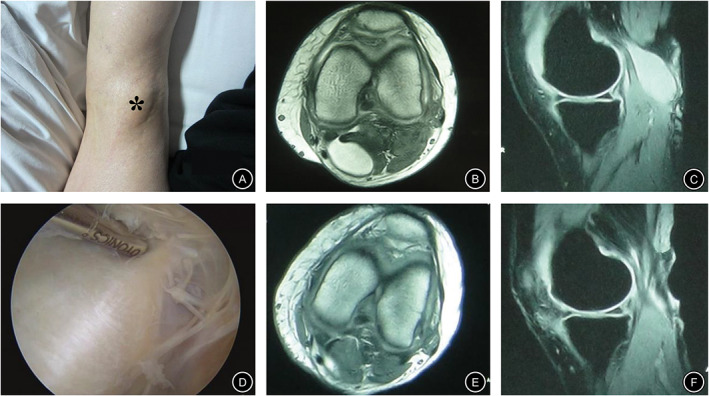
A 55‐year‐old male with posteromedial pain and distension of the right knee for more than 15 months and mild flexion limitation for 5 months. (A) Physical examination showing a cystic mass in the popliteal fossa (*). (B & C) A popliteal cyst identified by MRI on the right knee before surgery. According to R–L grade, the preoperative popliteal cyst was grade 3, but recovered to grade 0 following arthroscopic treatment. (D) Arthroscopic view of the cyst wall. (E & F) No recurrence of the cyst was detected by MRI after 28 months of follow‐up.
